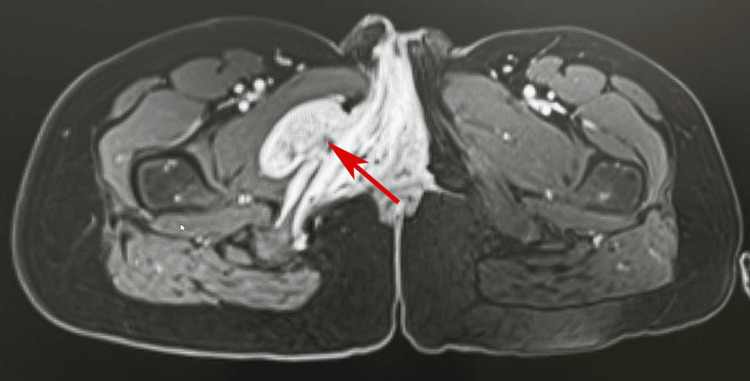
Magnetic resonance imaging with contrast shows unilateral right vulval enlargement containing a well-defined large mass measuring 7.3 × 5.3 × 1.6 cm (arrow).
